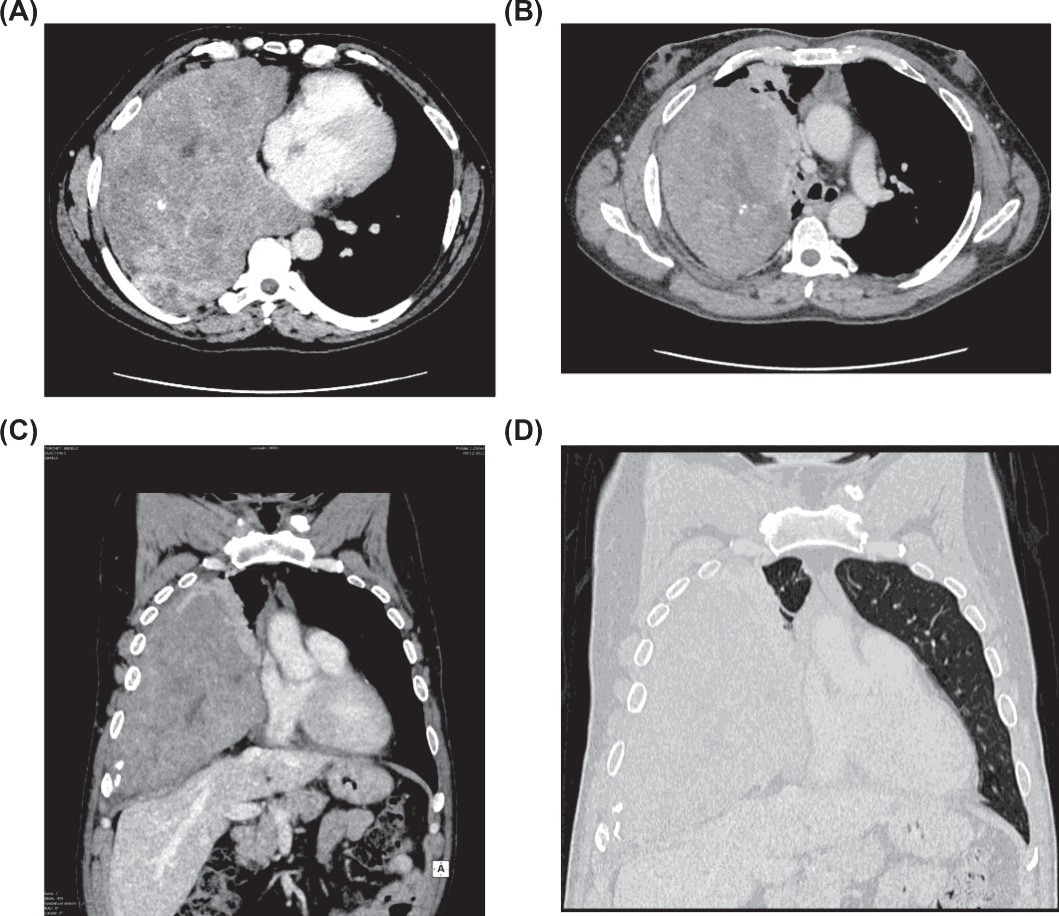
CT (computed tomography) and MPR (multiplanar reconstruction) images. (A, B) Large heterogeneously enhanced mass with superior vena cava and right atrium compression. (C, D) MPR: note parenchymal lung compression not involved right apical segment.
